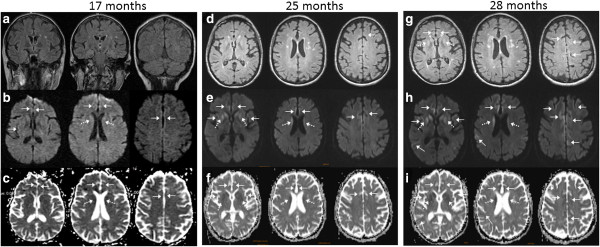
Serial brain MRIs, classic for CJD. Brain MRIs at 17 (a-c), 25 (d-f), and 28 (g-i) months. Solid arrows indicate cortical ribboning. Dotted arrows indicate deep nuclei hyperintensity. (a) Initial brain MRI was read by us as “ambiguous” for CJD, but was felt to show mild frontal-parietal atrophy and punctate subcortical white matter hyperintensities on FLAIR (b) DWI shows subtle cortical ribboning in the anterior and median cingulate and right posterior cingulate and subtle striatal hyperintensity. (c) ADC map shows hypointensities in the same regions as on DWI. (d) FLAIR (axial) brain MRI at 25 months reveals moderate diffuse cortical atrophy. (e) DWI now shows cortical ribboning in the right frontal cortex, and anterior and median cingulate, and hyperintensities in bilateral caudate and putamen with anterior to posterior gradient. (f) ADC map shows hypointensities in the areas of DWI hyperintensity. (g) FLAIR MRI at 28 months shows more prominent cingulate cortical ribboning hyperintensity in the striatum. (h) Cortical ribboning is more prominent than three months prior. (i) ADC map shows hypointensities consistent with restricted diffusion.
